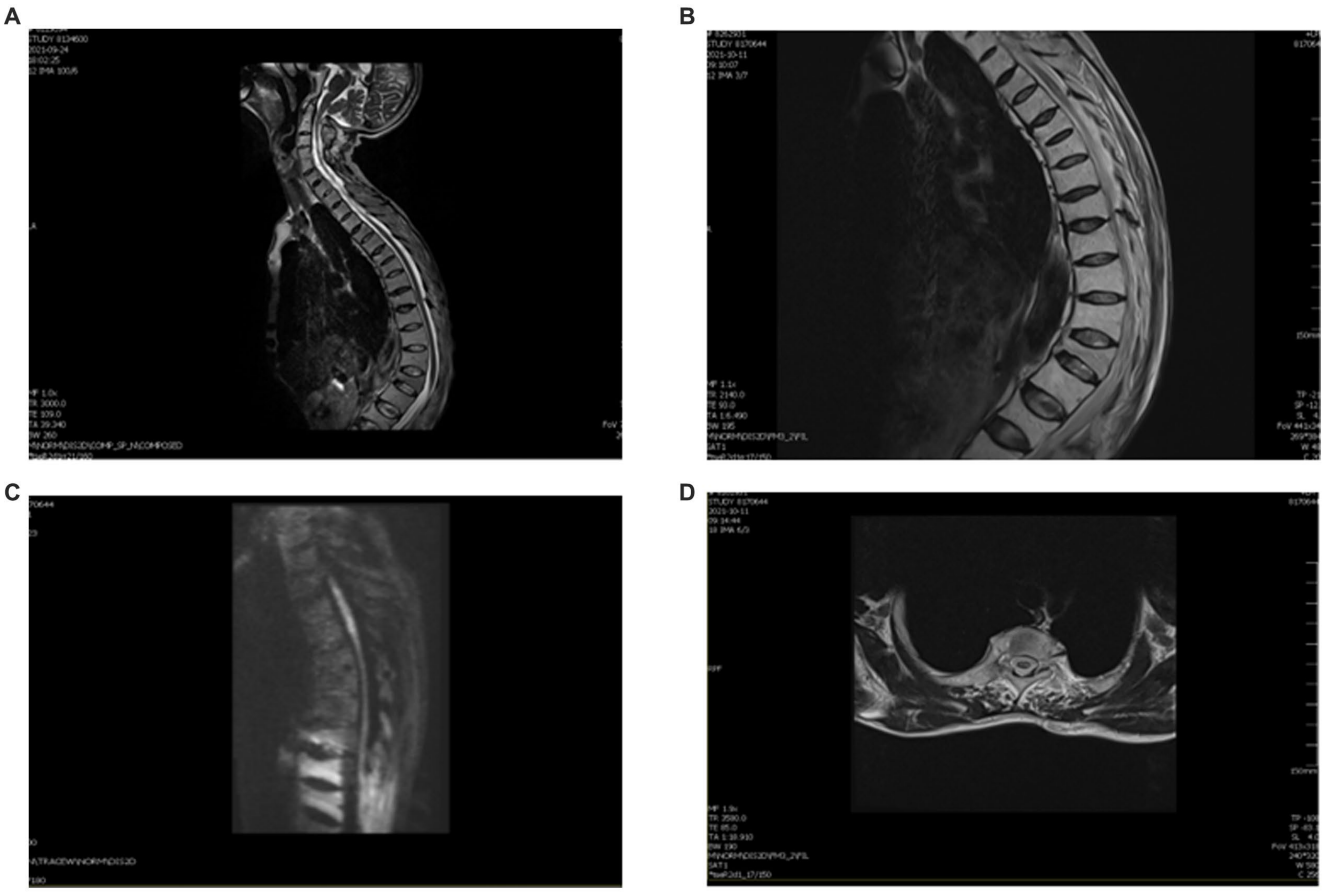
MRI of this patient after therapy. (A) Thoracic kyphosis with multiple vertebral wedgies at T4, T6, T7, T9, L1, and L3. (B) Sagittal T2 thoracic vertebrae T2–T5 are slightly hypersignal. (C) DWI shows a slightly elevated signal in the gray matter. (D) Axial T2 central hypersignal is less than before.
